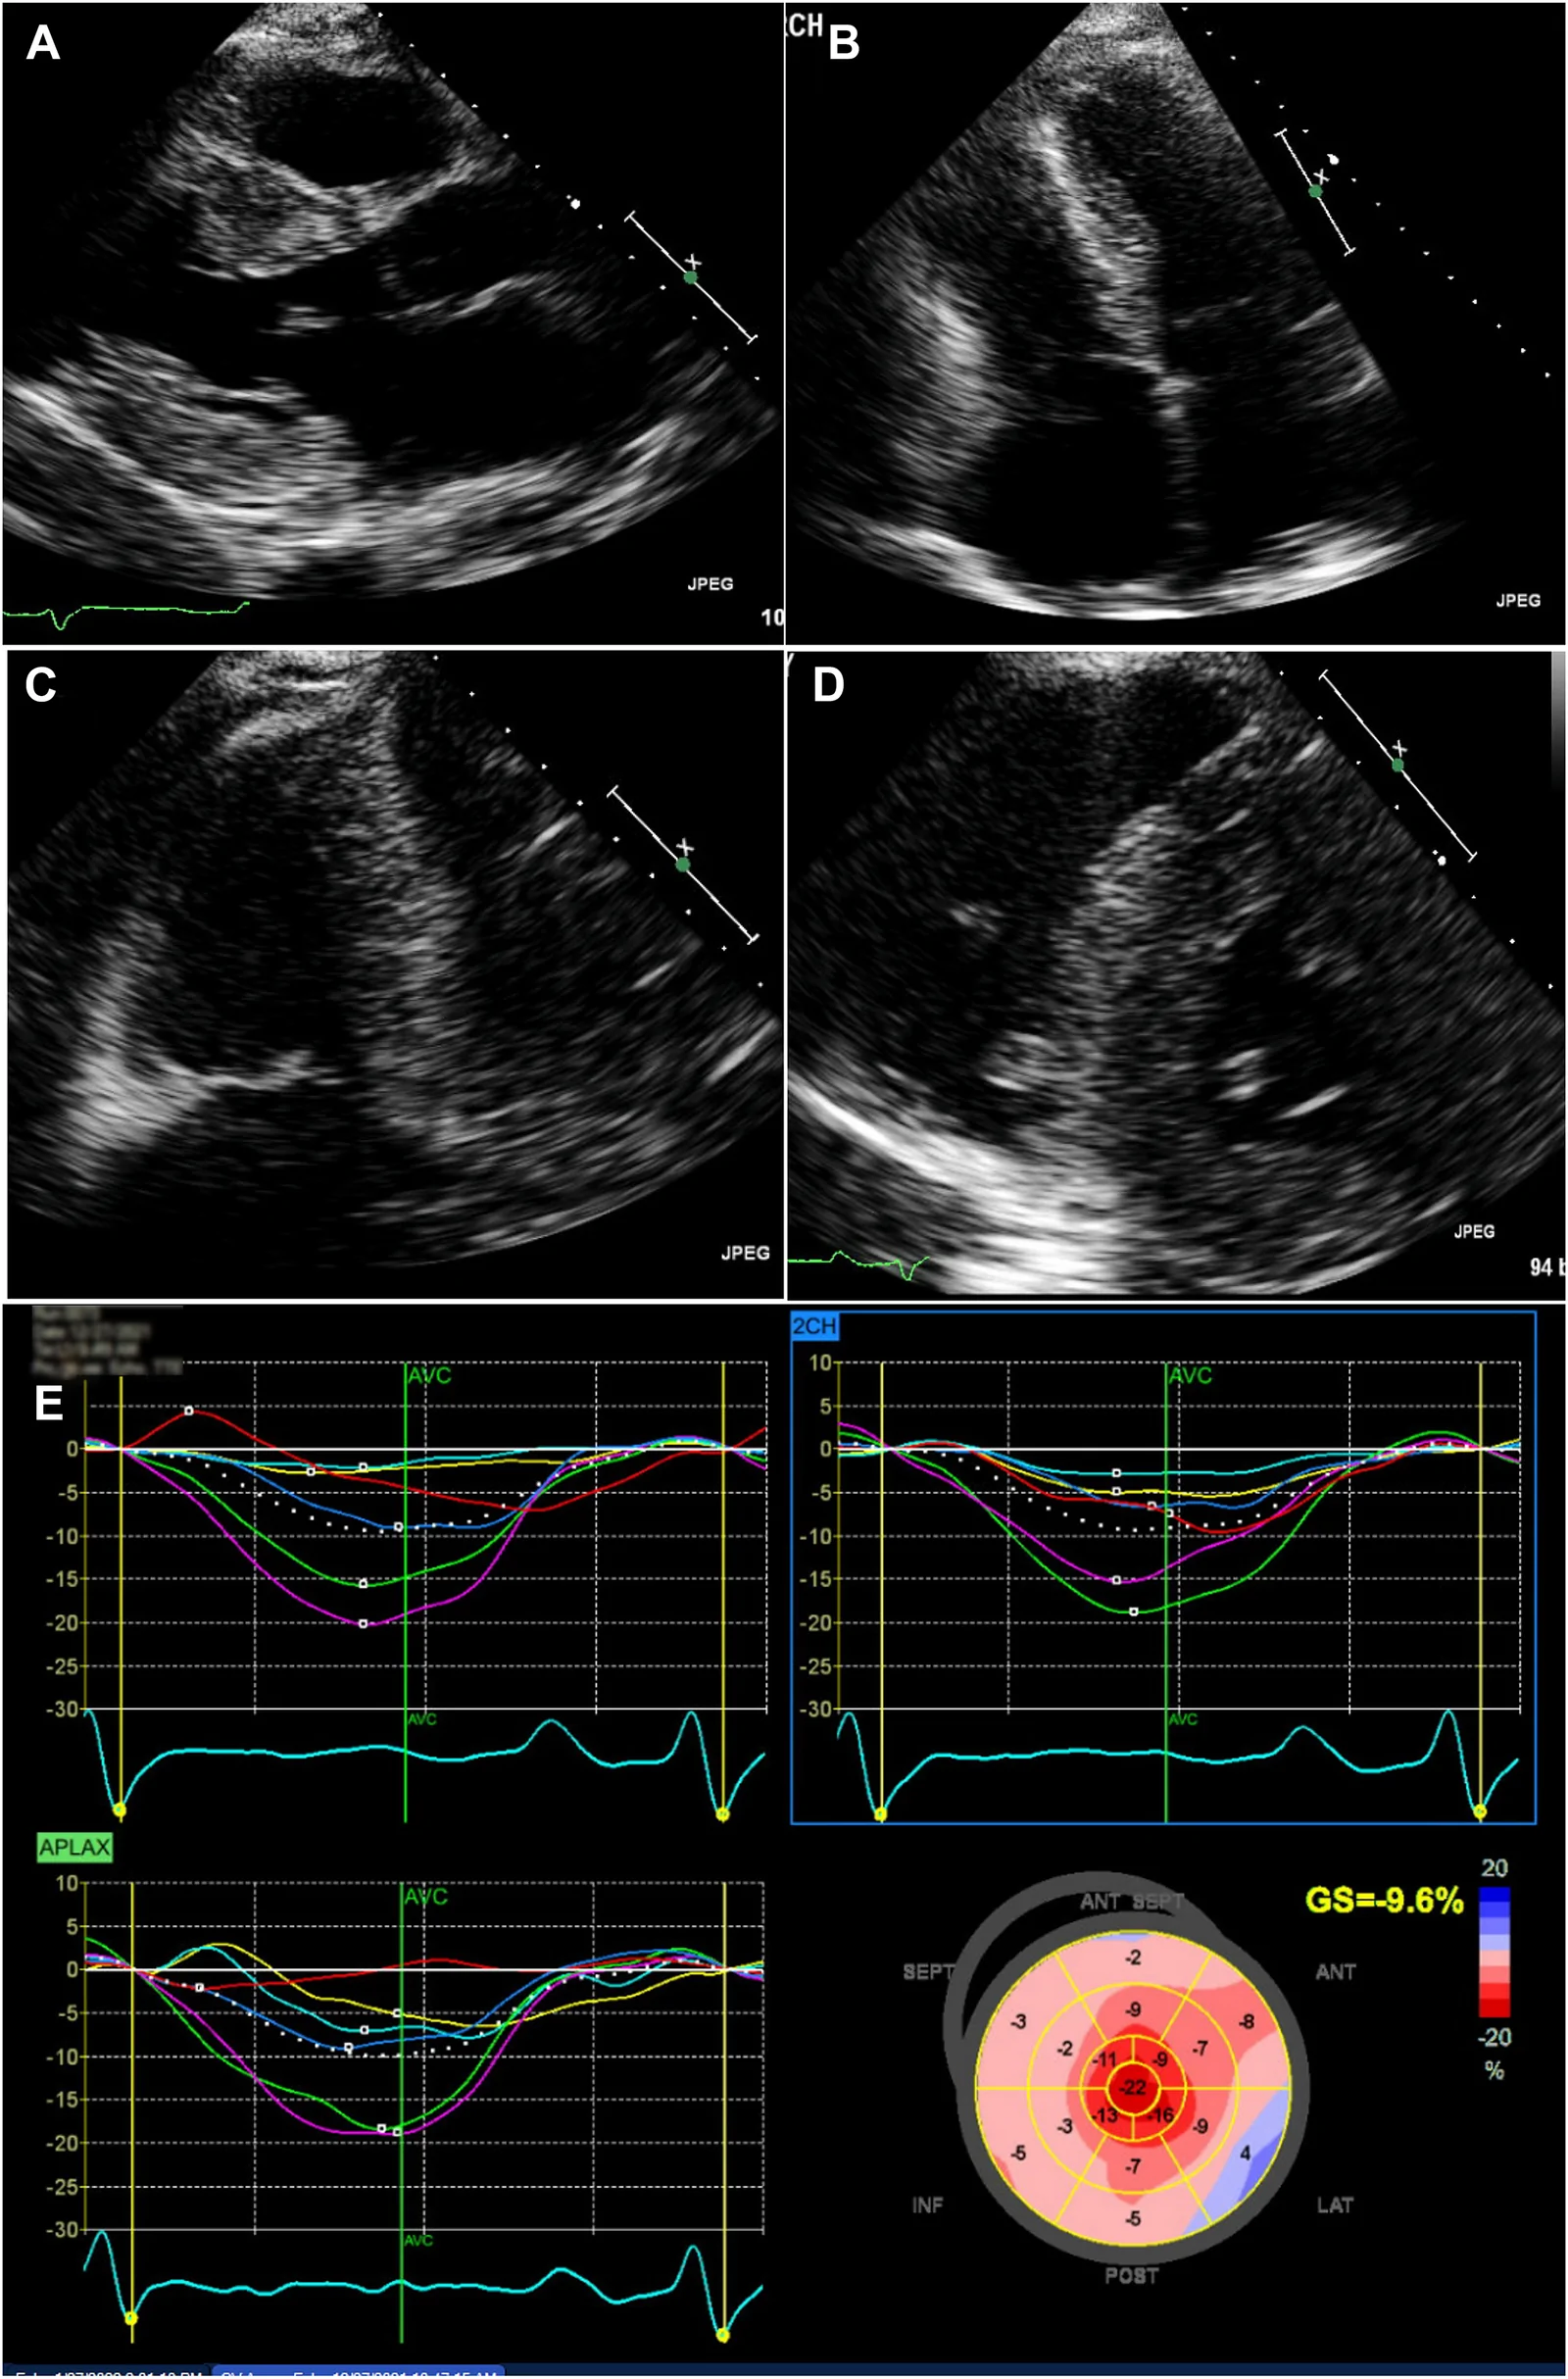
Two-Dimensional Echocardiogram (A) Parasternal long-axis view showing concentric left ventricular thickening. (B) Apical 4-chamber view showing biatrial enlargement. (C) Right ventricle–focused view showing mild right ventricular dilation. (D) Parasternal short-axis view showing left ventricular thickening. (E) Strain imaging showing apical sparing.
